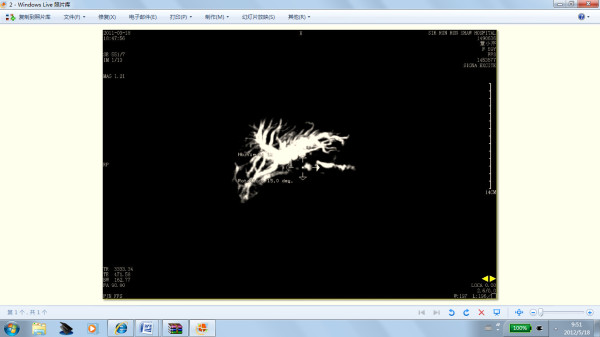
MRI showed pancreatic duct was dilated after surgery during follow-up
